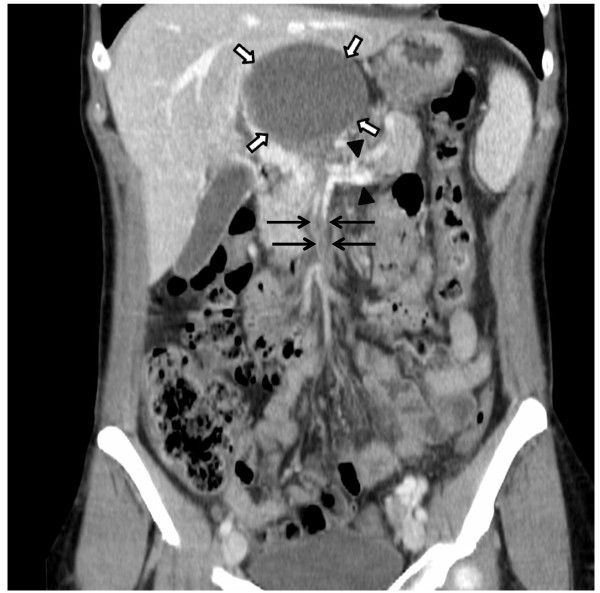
CT-scan showing decreasing size of thrombus within portal vein aneurysm (white arrows) with diminished extension to SMV (black arrows) and SV (arrowheads).
